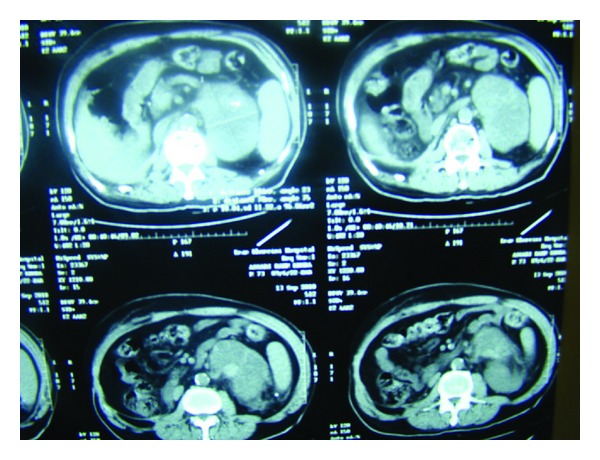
Contrast enhanced abdominal CT scan, revealing the left renal mass.
